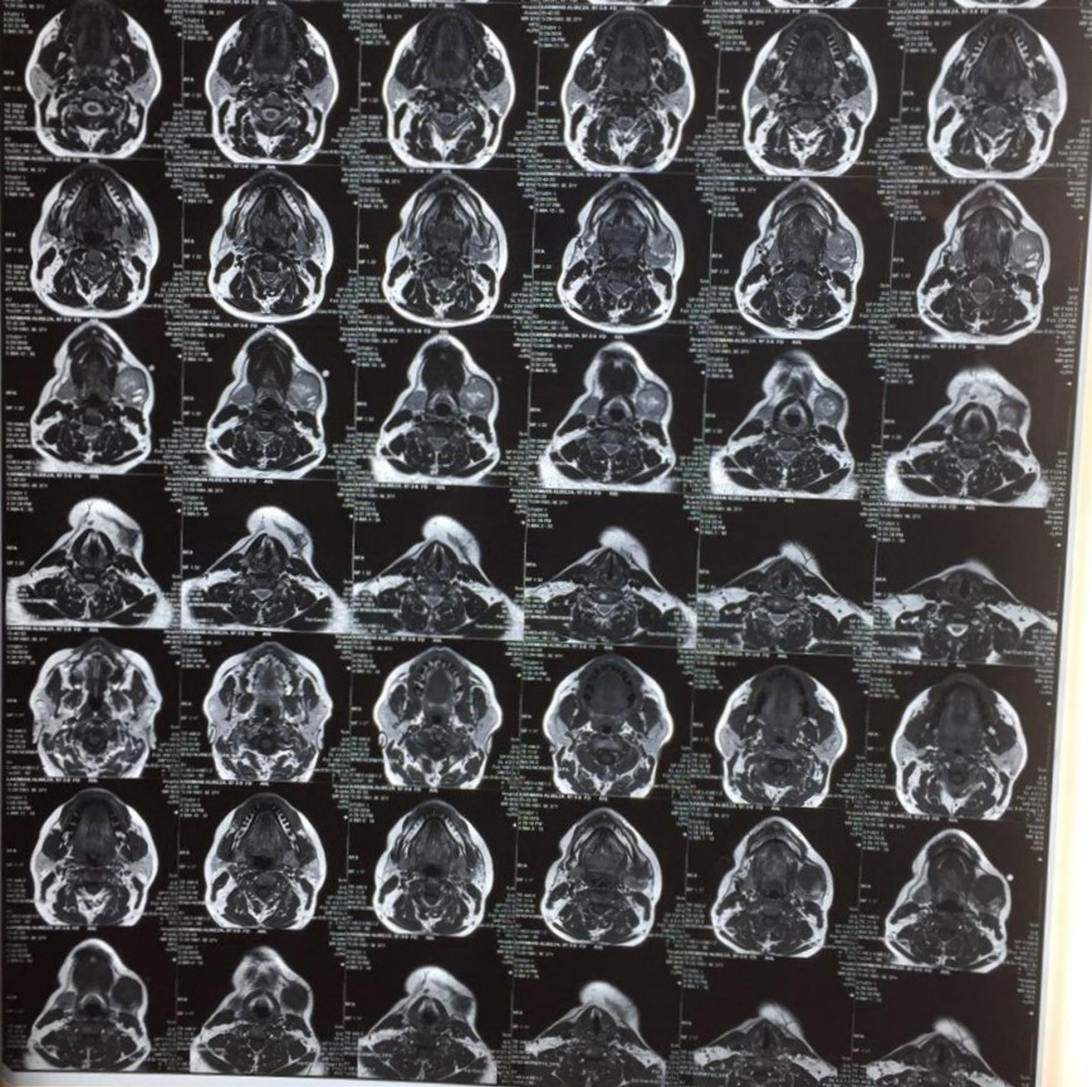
Sections of T1 Weighted MRI. There was evidence of a 28 × 24 mm large heterogenic mass in the left submandibular space
